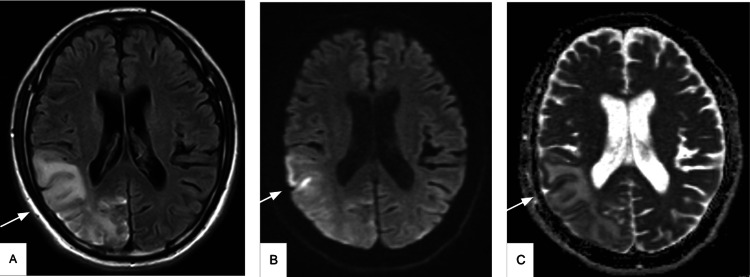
Preoperative MRI brain Gyriform cortical diffusion restriction involving the right temporoparietal and occipital regions but sparing the subjacent subcortical white matter (white arrows), suggesting tissue injury in a nonvascular distribution. A. T2-weighted fluid-attenuated inversion recovery (FLAIR). B. Diffusion-weighted imaging (DWI). C. Apparent diffusion coefficient (ADC).
